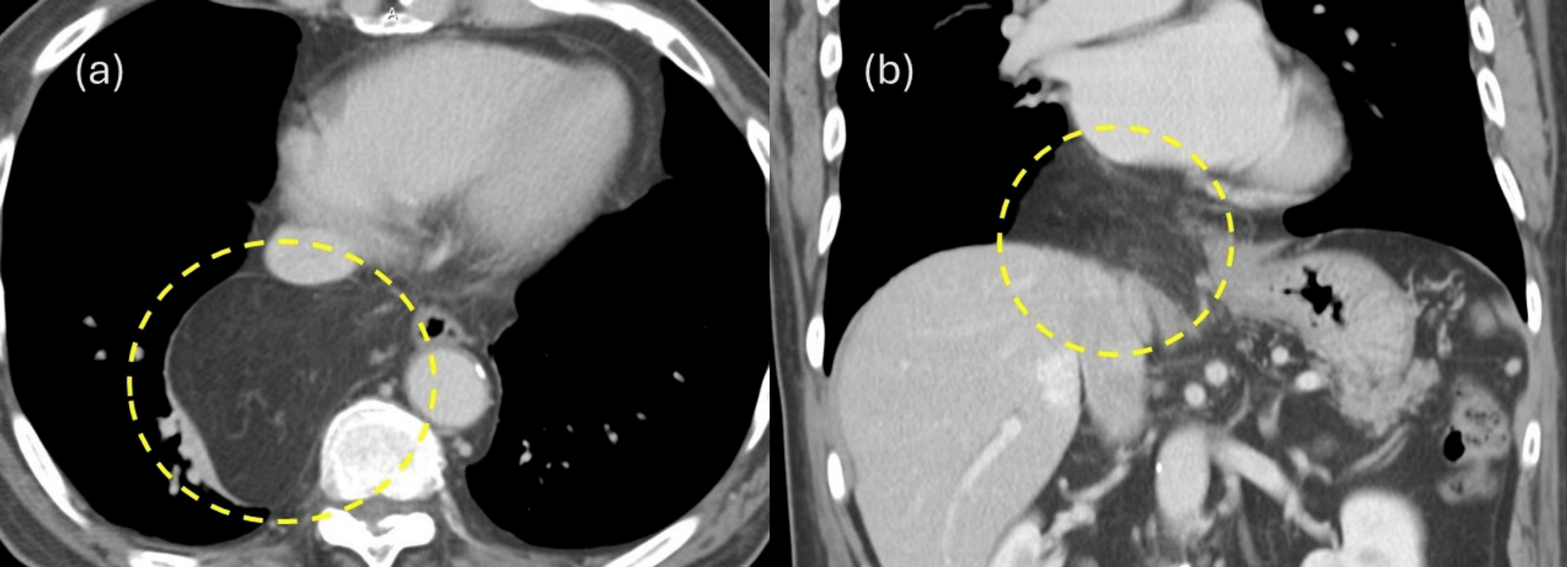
Contrast-enhanced CT Intraabdominal fat was found to be prolapsed into the mediastinum via the esophageal hiatus
